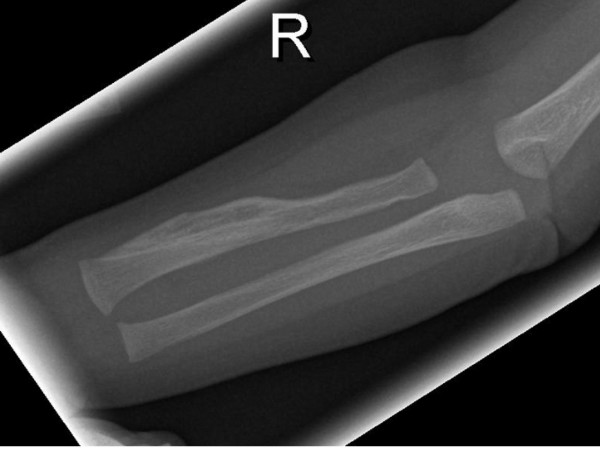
Anteroposterior radiograph of the radius showed cortical new bone formation associated with subperiosteal thickening. Note marked bloating along the diaphysis with sparing of the epi-metaphyseal components associated with expansion of the bone marrow cavity and a persistent-like deformity (fig 4).
